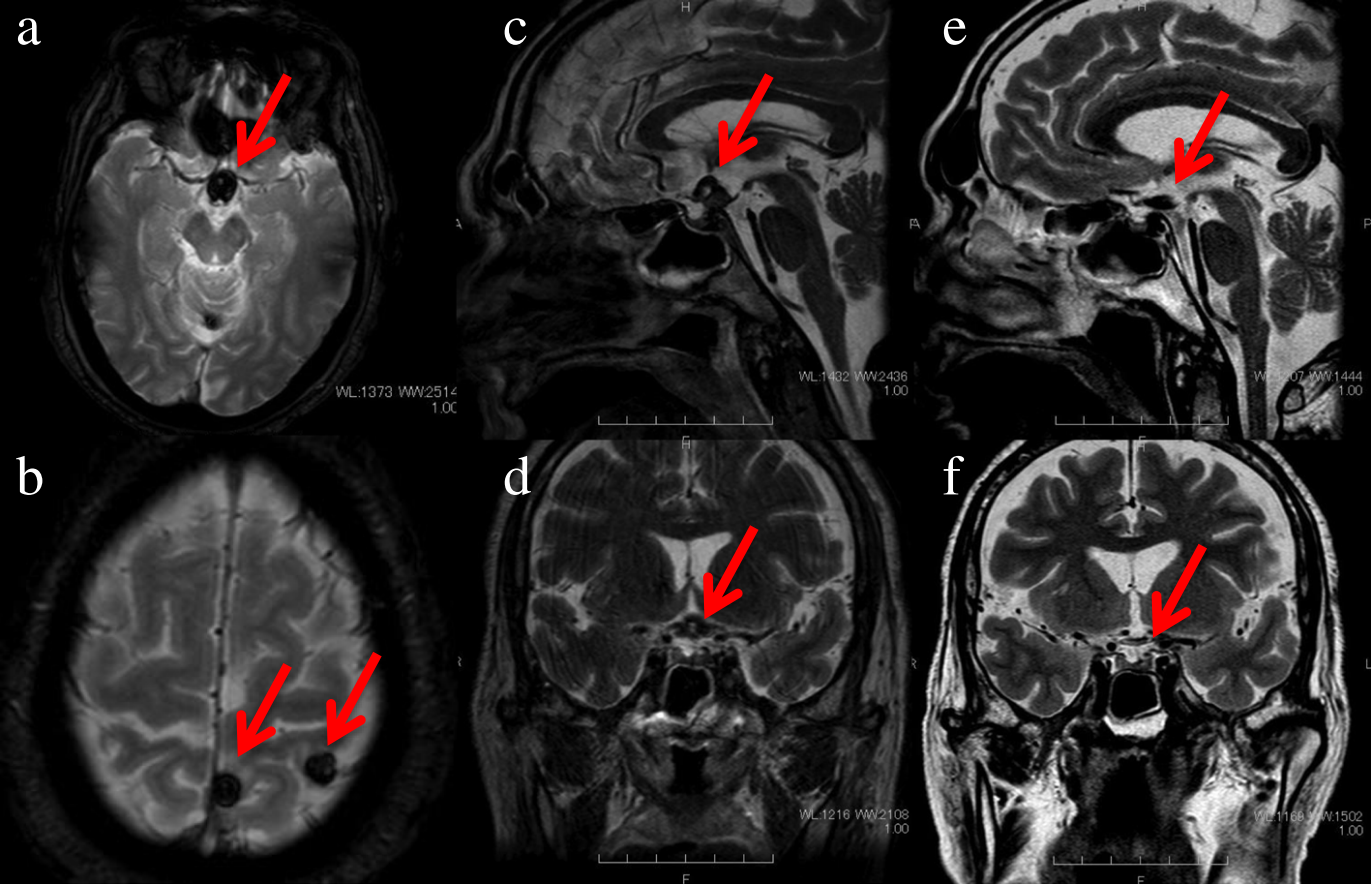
Brain magnetic resonance imaging (MRI) in T2 star-weighted sequences revealing multiple masses (a, b) and a 10-mm mass on the recessus infundibulum (c, d). On day 62, brain MRI in T2 star-weighted sequences demonstrated the disappearance of the suprasellar tumor (e, f) The arrows are pointing to multiple masses
